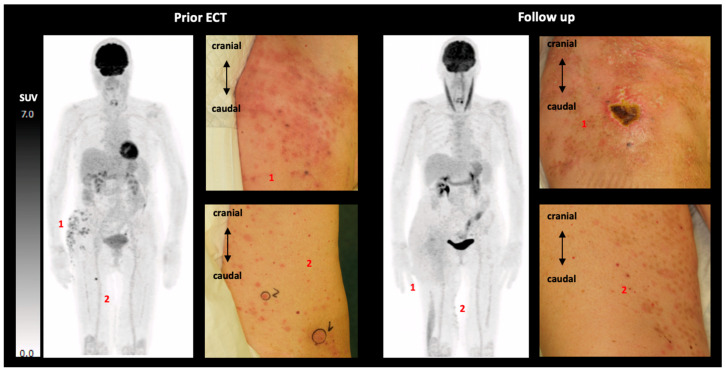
FDG PET imaging and clinical observations pre-ECT and at follow-up. The maximum intensity projection (MIP) prior to ECT revealed disseminated melanoma lesions at the right hip (1) and inner upper thigh (2) with a maximum SUV_max_ of 6.2, which was consistent with clinical observations (Pat. ID 4). However, metastases at the inner upper thigh showed only limited FDG uptake on PET. In the subsequent evaluation conducted approximately two months following the ECT, the presence of new lesions was identified on the PET scan, particularly in the inner upper thigh region (CT: maximum diameter 0.5 cm; PET: maximum SUV_max_ 2.0). However, progression was predominantly observed through clinical observation. ECT electrochemotherapy; MIP maximum intensity projection.
